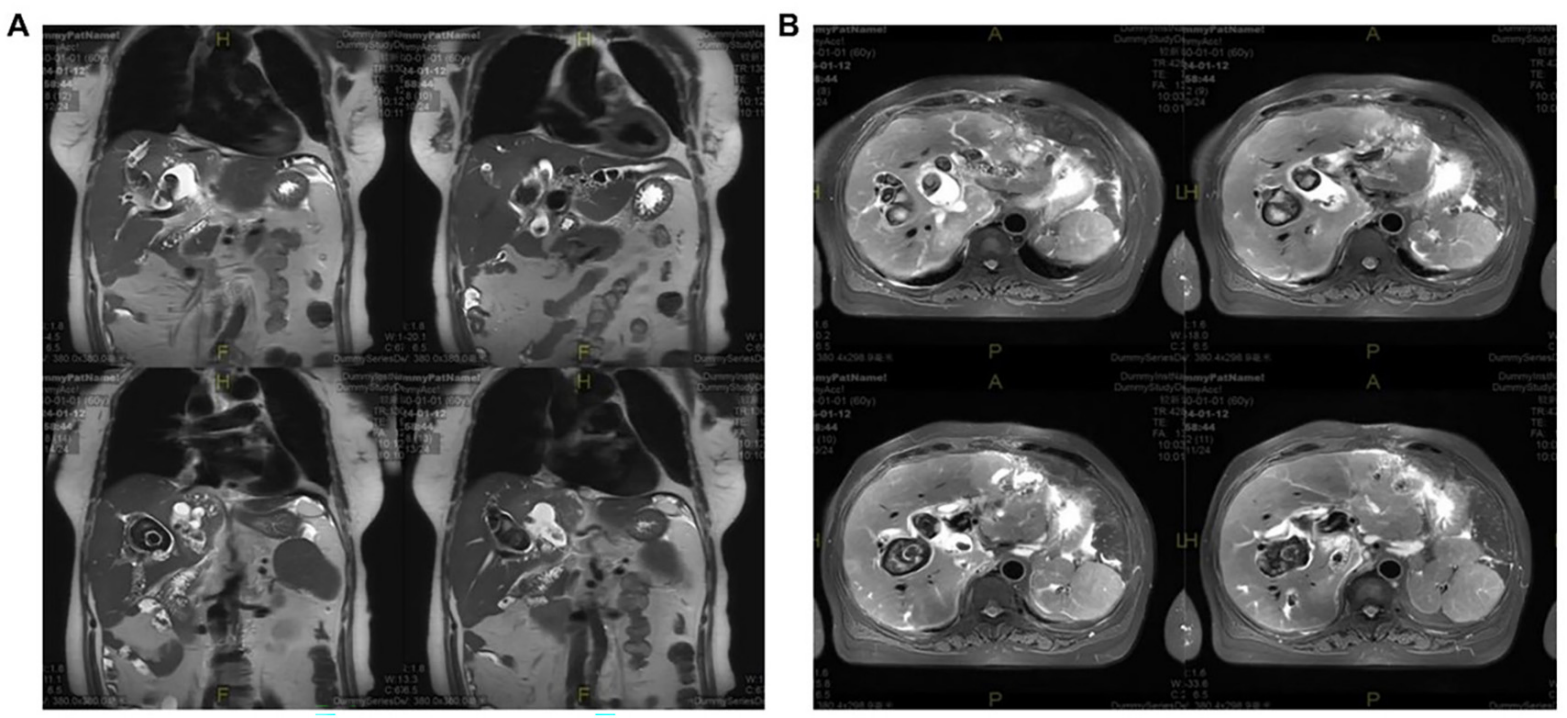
MRI images demonstrating multiple bile duct stones in panel (A) coronal and (B) axial planes.
